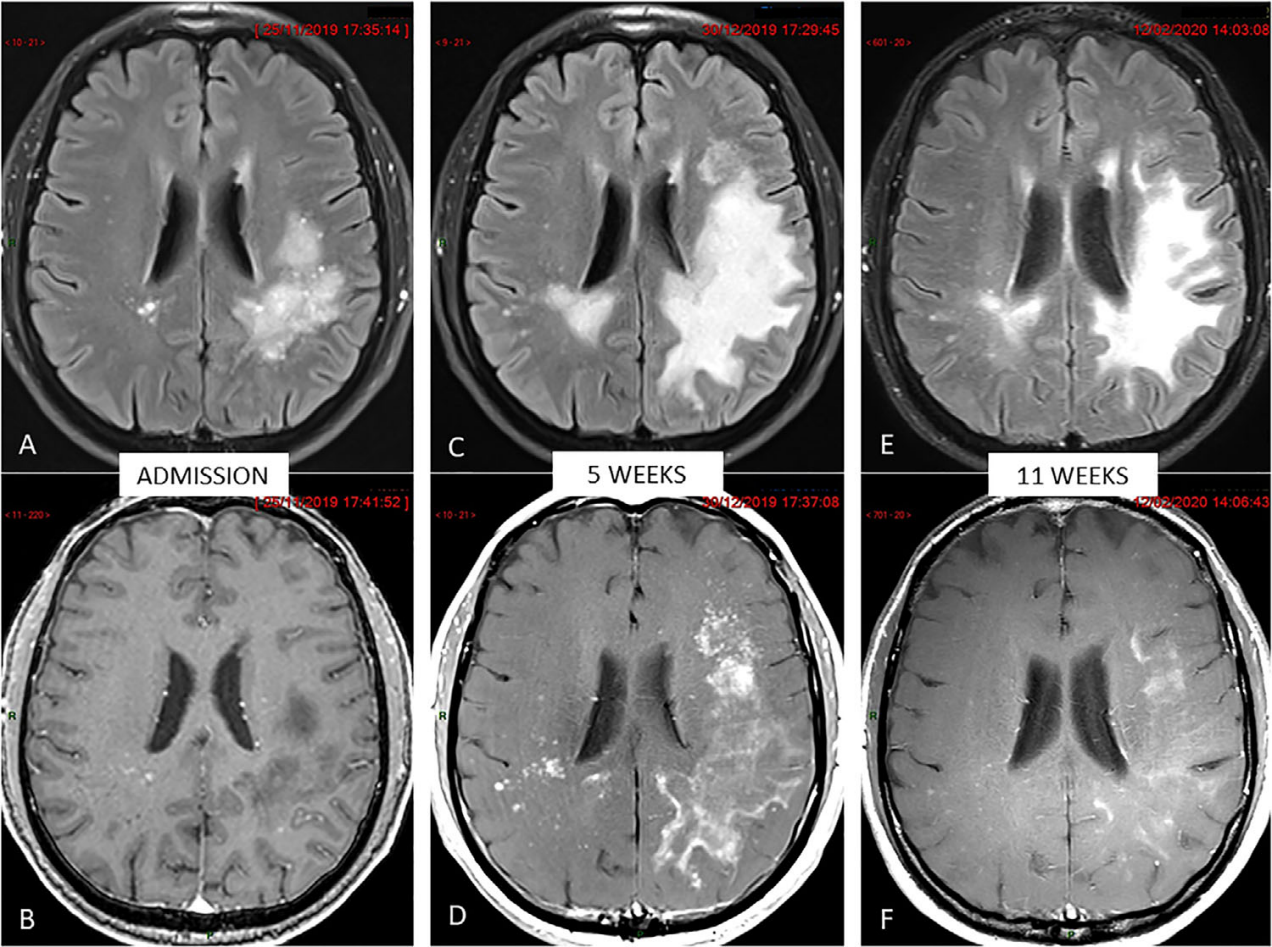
Brain magnetic resonance monitoring (all images in similar slice location). Upper row: Contrast‐enhanced FLAIR (fluid‐attenuated inversion recovery) views. Lower row: Contrast‐enhanced T1‐weighted views. A and B, Pretreatment baseline examination showing bilateral but asymmetric hyperintense changes in the parietal white matter on FLAIR image (A) appearing hypointense with only a few enhanced foci on post‐contrast image (B). C and D, Follow‐up examination 5 weeks later showing increase in size of damaged parenchyma on FLAIR image (C) together with dramatic increase of contrast‐enhancement featuring immune restoration (D). E and F, Follow‐up examination 11 weeks later showing moderate subsidence of FLAIR abnormalities (E) but strong decrease of areas of contrast enhancement (F) featuring the restoration of a competent brain‐blood barrier (BBB) after the inflammatory blaze seen in (D)
